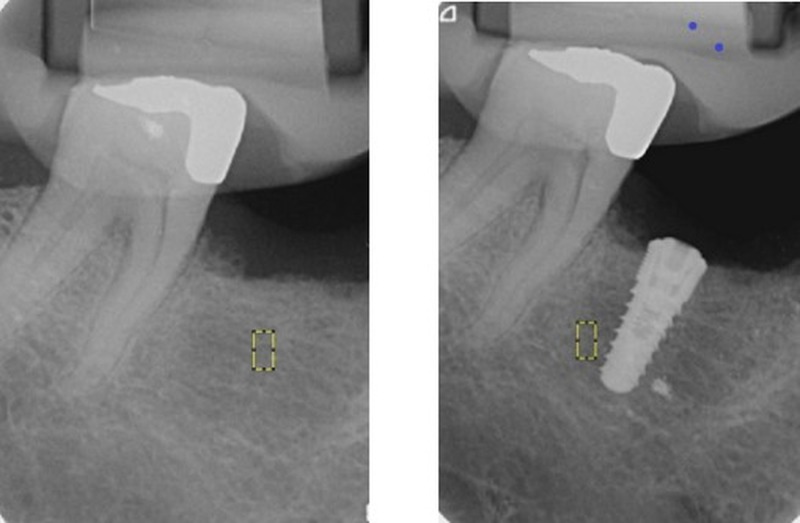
Region of interest at the recipient site on the preoperative radiograph and adjacent to the inserted implant immediately on postoperative radiograph - Preoperative periapical radiograph (before the insertion of the implant): a region of interest is created and set to a width of 25 pixels and a height of 50 pixels. The fractal dimension is then measured at this site. - Postoperative periapical radiograph (after the insertion of the implant): a region of interest is created with the same dimensions as those on the preoperative radiograph, adjacent to the inserted implant area. The fractal dimension is also measured at this site.
